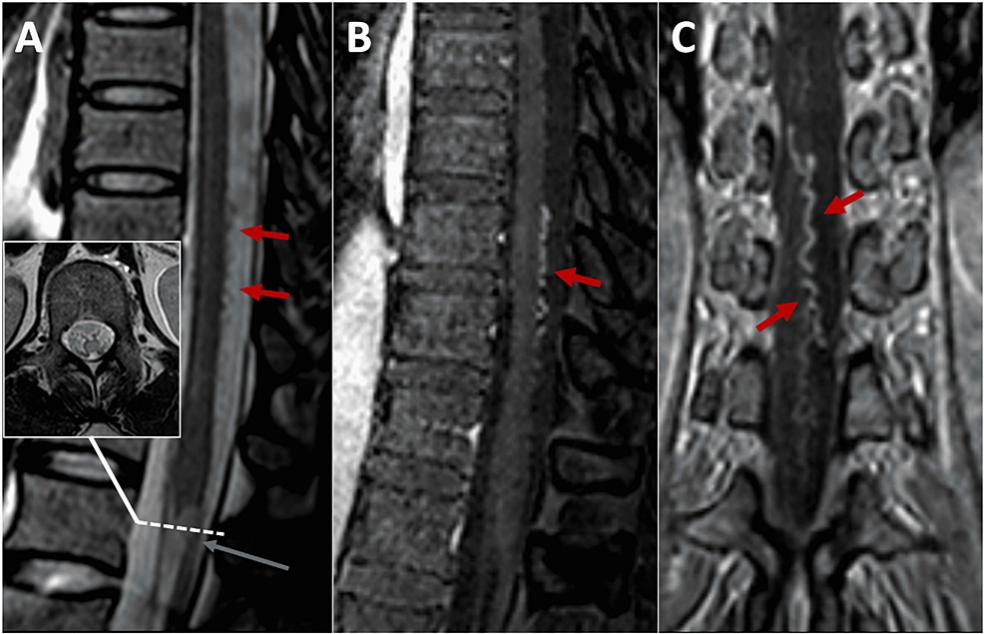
Spinal cord imaging of case 1. (A) T2-weighted sagittal image of the thoracic spine where diffuse hyperintensity is identified in the medullary cone (gray arrow in A), which is confirmed in the axial image. In addition, the flow-void serpentine image on the posterior surface of the spinal cord (red arrows in A). (B) Sagittal and (C) coronal angiographic images showing dilated and tortuous structures on the posterior surface of the spinal cord.
